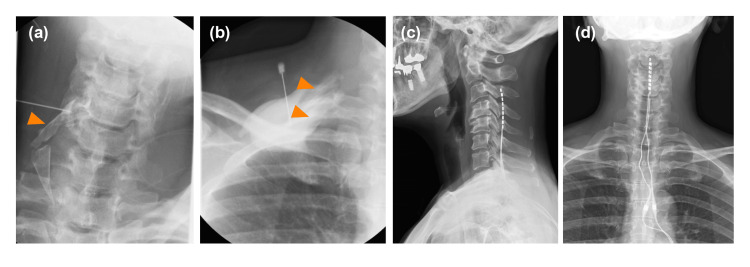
Peripheral nerve block and spinal cord stimulation. (a) C6 pulsed radiofrequency: The arrow indicates the contrasted right sixth cervical nerve root. (b) Brachial plexus block: The arrows indicate the contrasted anterior and middle scalene muscles. (c) Lateral radiographic image of spinal cord electrodes. (d) Frontal radiographic image of spinal cord electrodes.
